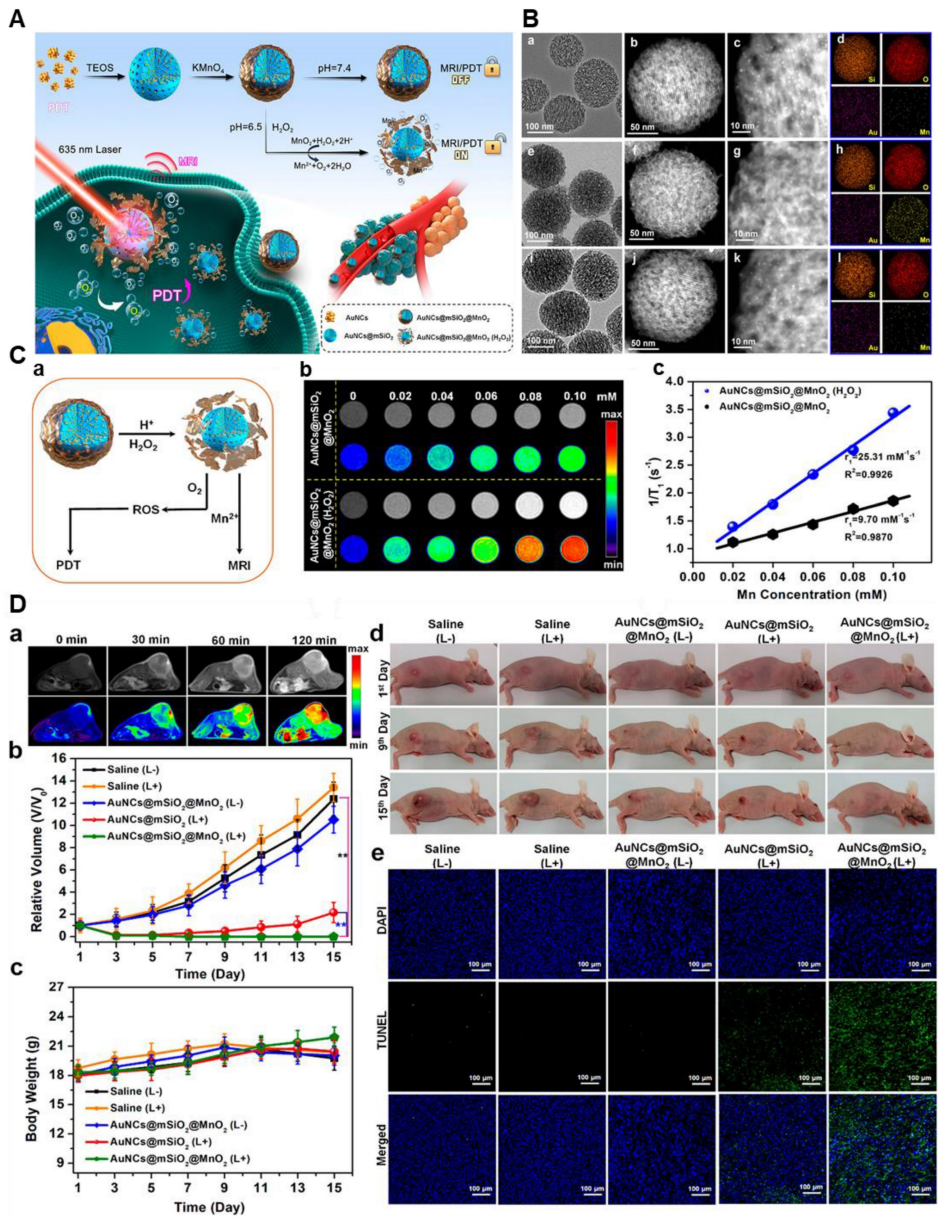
(A) AuNCs@mSiO_2_@MnO_2_ nanozyme synthesis process for H_2_O_2_-responsive "Off/On" regulation and upgrade of MR imaging and photodynamic therapy. (B) TEM, STEM, high-amplification STEM, and component planning pictures of the synthesized samples. (a-d) AuNCs@mSiO_2_; (e-h) AuNCs@mSiO_2_@MnO_2_; and (I-l) AuNCs@mSiO_2_ @MnO_2_ (H_2_O_2_). (C) H_2_O_2_ -responsive MR, O_2_, and ^1^O_2_ age execution. (a) "Off/on" tweak and upgrade of MR imaging, PDT; (c) *T1* - weighted MR imaging; (d) longitudinal MR unwinding bends of samples. (D)
